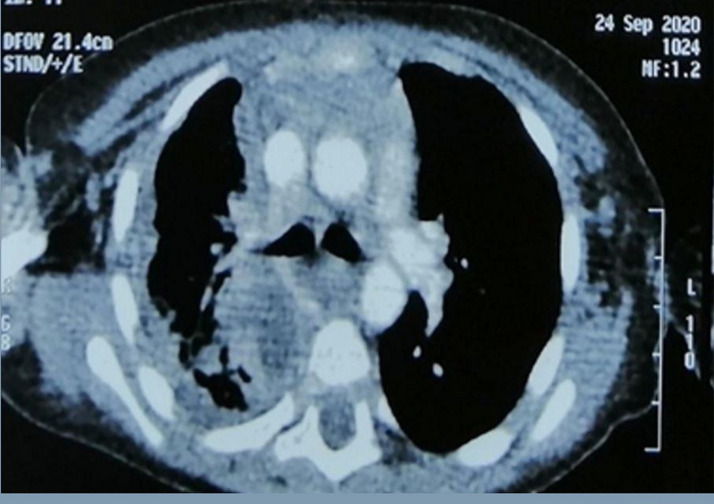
the control thoracic CT scan showing regression of the mediastinal collection and of the pleural empyema
